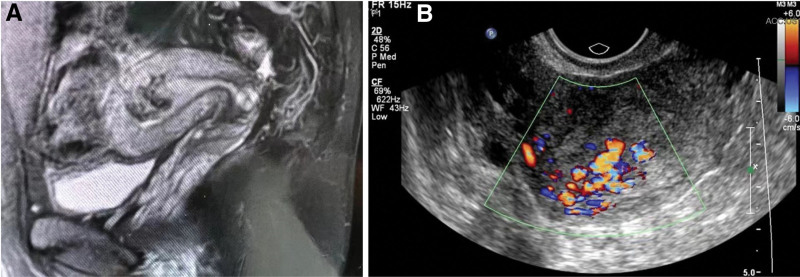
Pelvic MRI (A) and TVS (B) show a UAVF of the right posterior wall measuring 3.6 × 2.6 × 2.1 cm (case 1). MRI = magnetic resonance imaging, TVS = transvaginal ultrasonography, UAVF = uterine arteriovenous fistula.
